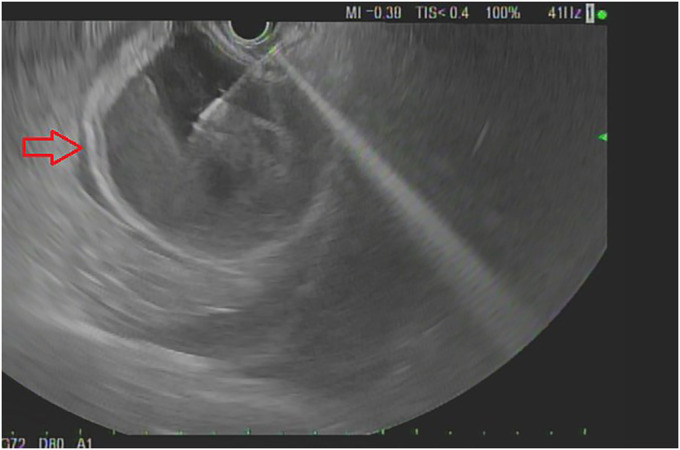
Endoscopic ultrasound (EUS) images revealing the wall layers of the cystic structure (thick arrow) is seen after cyst fluid fine-needle aspiration (FNA), which demonstrates that it is not a cyst but a gastrointestinal structure.
